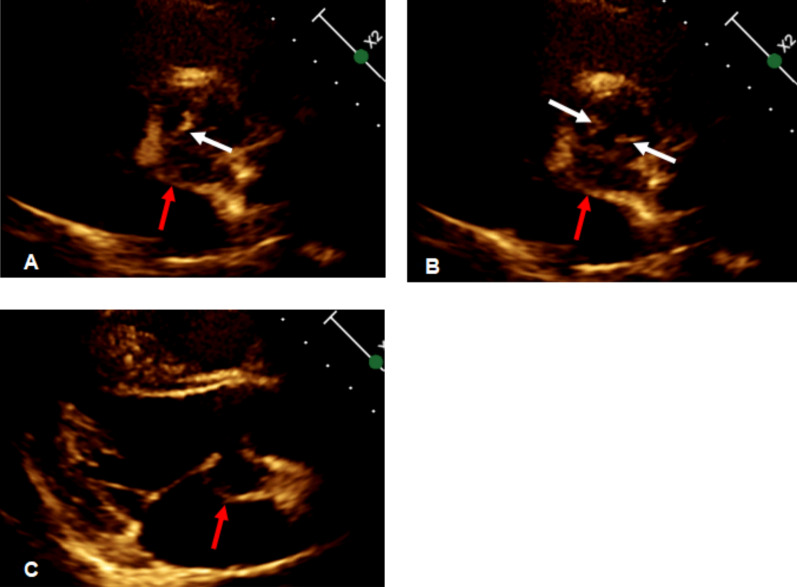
Transthoracic echocardiography (parasternal long-axis view). A, B Systolic and diastolic phases showing the bicuspid aortic valve. C An echolucent space (red arrow) is visible between the aortic annulus and the anterior mitral leaflet, suspicious for pseudoaneurysm
